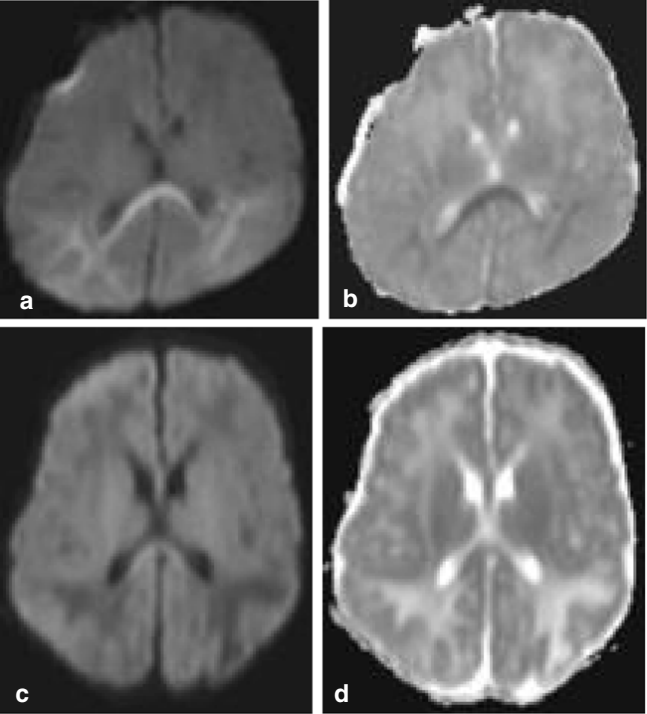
Seven-day-old child born at 38 + 5 weeks with a difficult delivery and an APGAR score of 4–5–6 was admitted to another hospital. It was transferred to our hospital because of seizures at 2 days postpartum. On admission, the child had a hypoglycemia (< 0.5 mmol/l) for which glucose infusion was given. DWI (a) and ADC (b) made 5 days after admission showed diffusion restriction in the splenium and in the subcortical white matter of the parietal-occipital lobes. On follow-up DWI (c) and ADC (d) made 16 days after admission, the signal intensity of the splenium normalized, with remaining white matter edema in the parietal lobes and widening of the ventricles, due to global tissueloss
